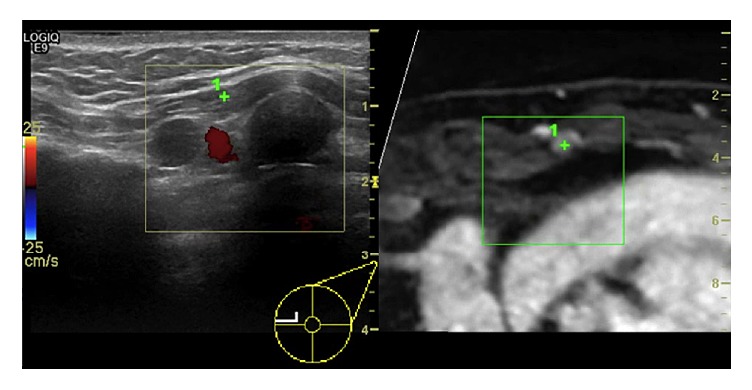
Ultrasound image (left side) with the corresponding multiplanar reconstructed MR image (right side) of a 55-year-old woman who underwent a previous surgery of the left breast for invasive ductal carcinoma (IDC, pT2N0). After 6 months, a follow-up MR was performed showing a rounded enhancing lesion in the left internal mammary chain (green cross). Second-look ultrasound with coregistration revealed a pathological lymph node.
